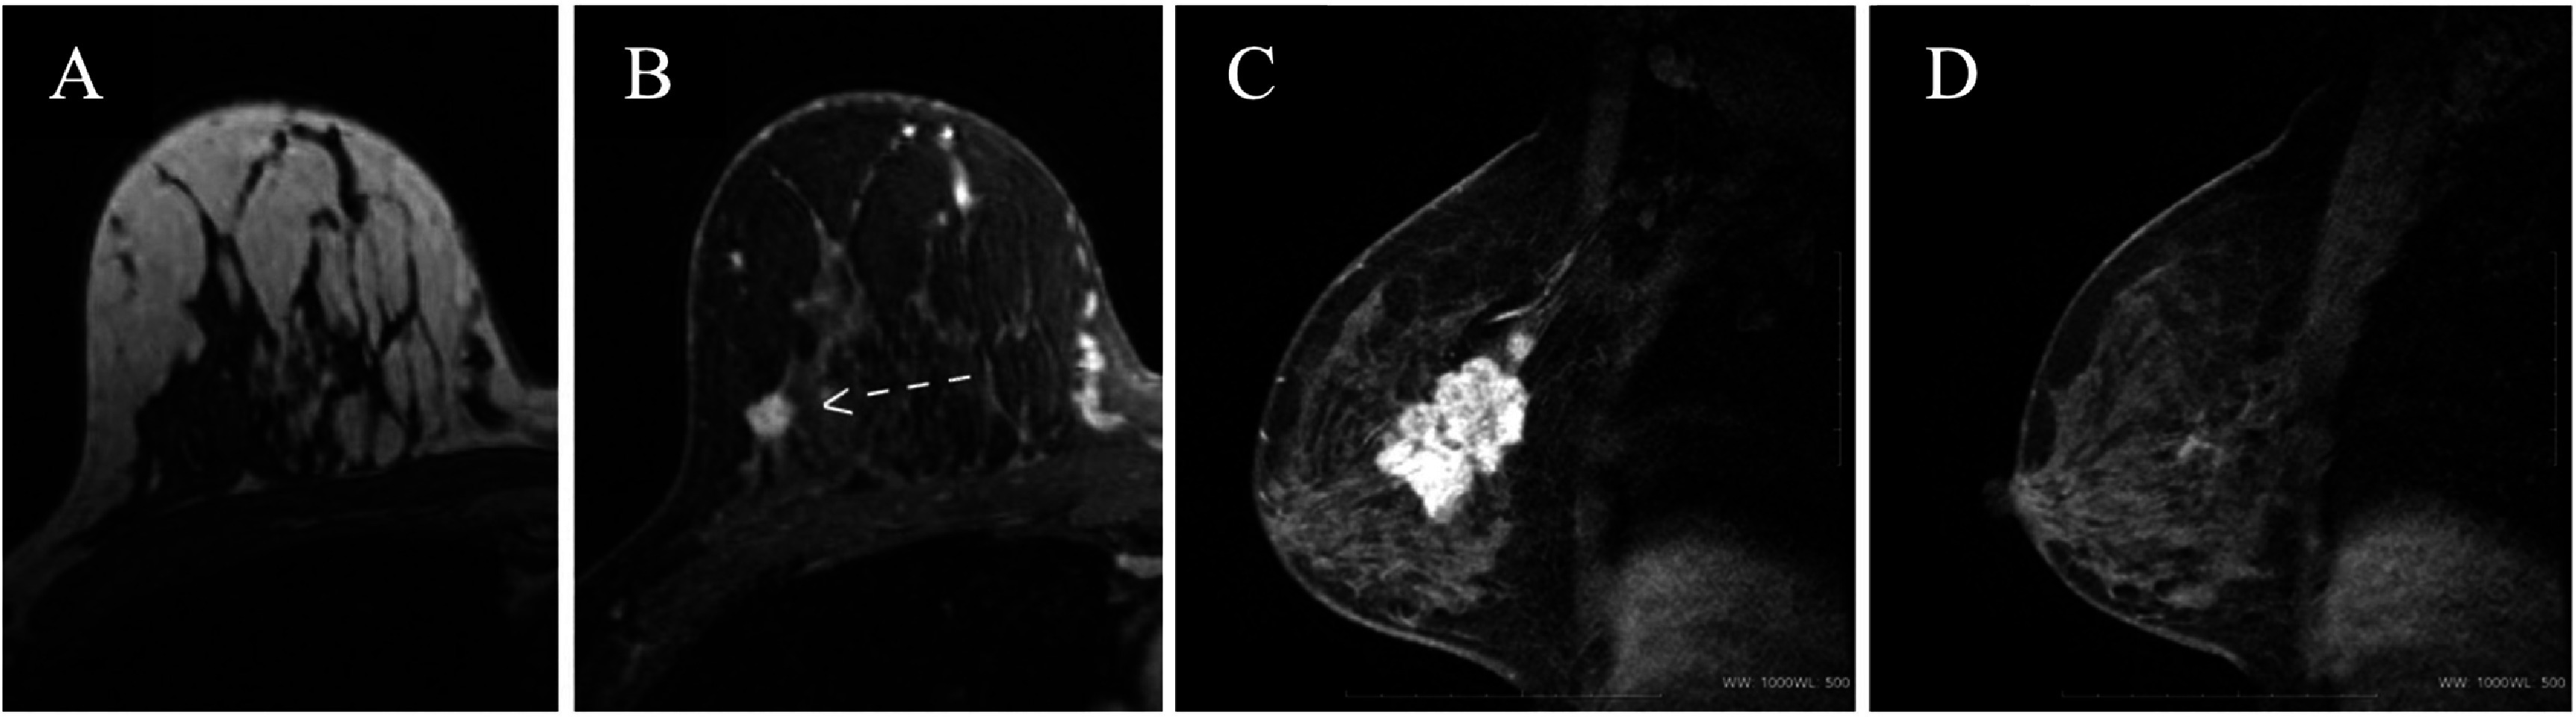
Breast cancer detection with MRI. (A) Cancer is difficult to see in the absence of a contrast agent. (B) Cancer is clear (arrow) when a contrast agent is used. A 53 yr-old female patient’s MRI images (with injection of Gd-DTPA) taken before (C) and after (D) NAC treatment demonstrate how the lesion decreased following treatment. (A) and (B) Reprinted from [123], Copyright (2005), with permission from Elsevier. (C) and (D) Reprinted from [124], Copyright (2015), with permission from Elsevier.
